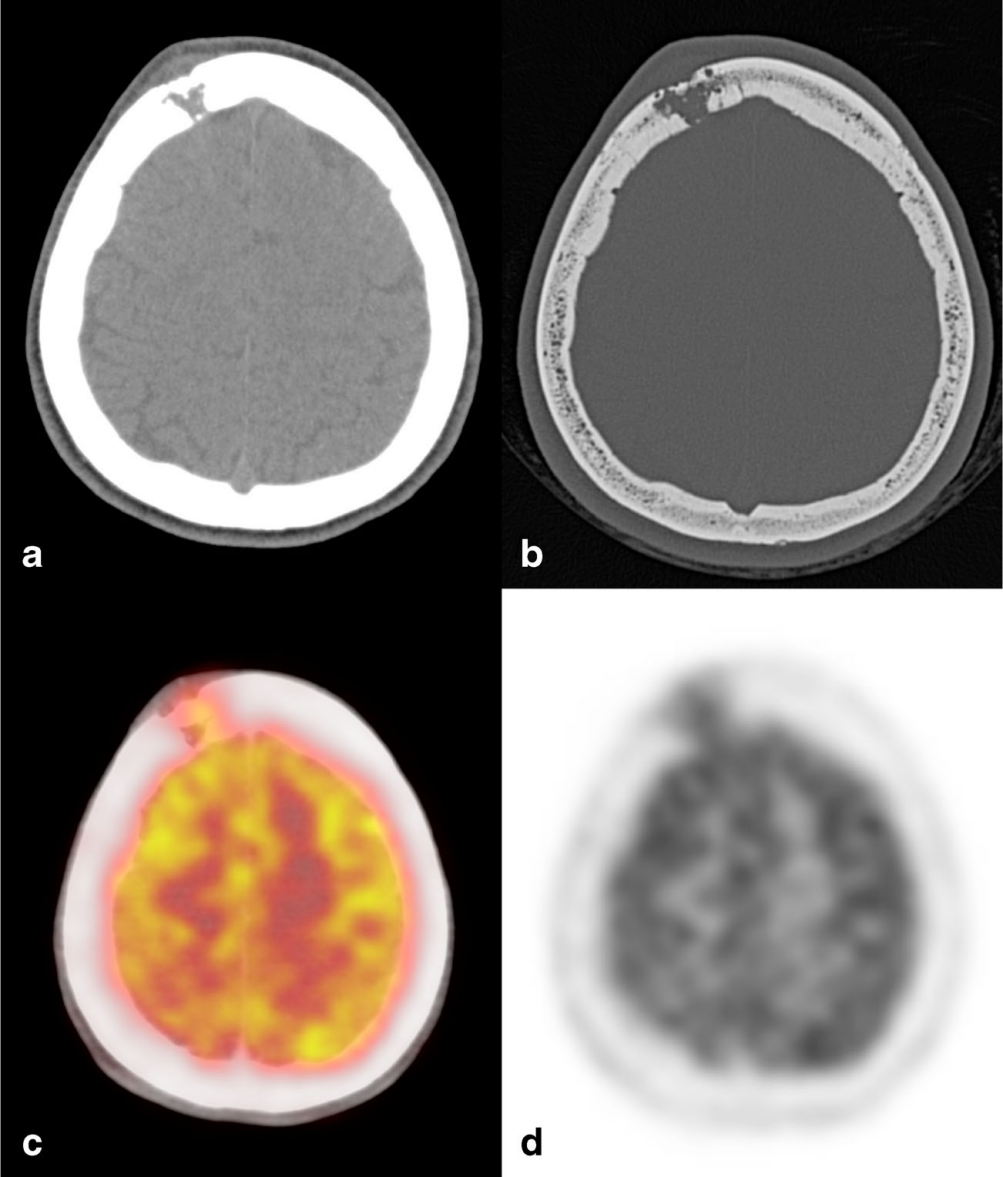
Eosinophilic granuloma (EG). Axial CT images with soft tissues (a) and bone (b) windows showing a right frontal lytic lesion with ill-defined margins extending in the contiguous extracranial soft tissues. Erosion of the inner cranial table is more pronounced than of the outer (“hole within a hole” sign). 18(F) FDG-PET CT examination demonstrates a moderate tracer uptake (c, d)
